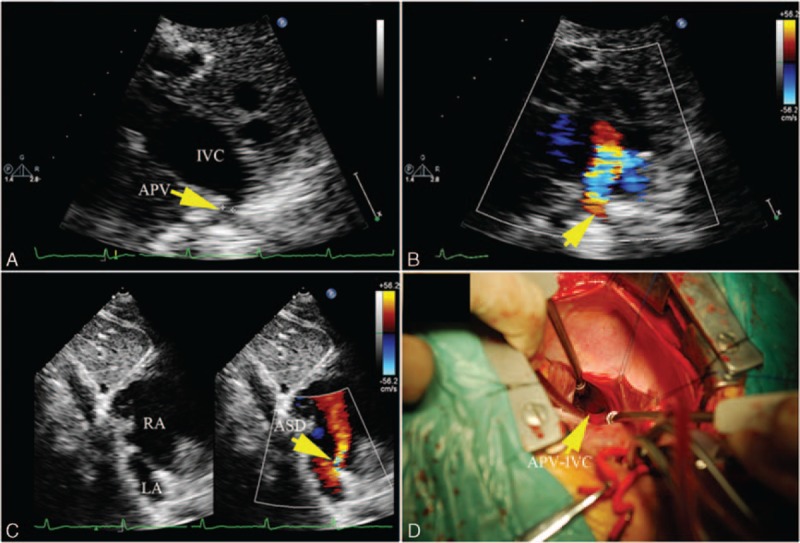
A 24-year-old girl with right pulmonary vein drainage into the inferior vena cava (IVC). (A) Subcostal view shows a tube drainage to IVC. (B) Color Doppler shows the abnormal flow spray into the IVC. (C) 2-dimensional and color Doppler showing asecundum atrial septal defect. (D) Intraoperative image shows an anomalous opening to the IVC.
